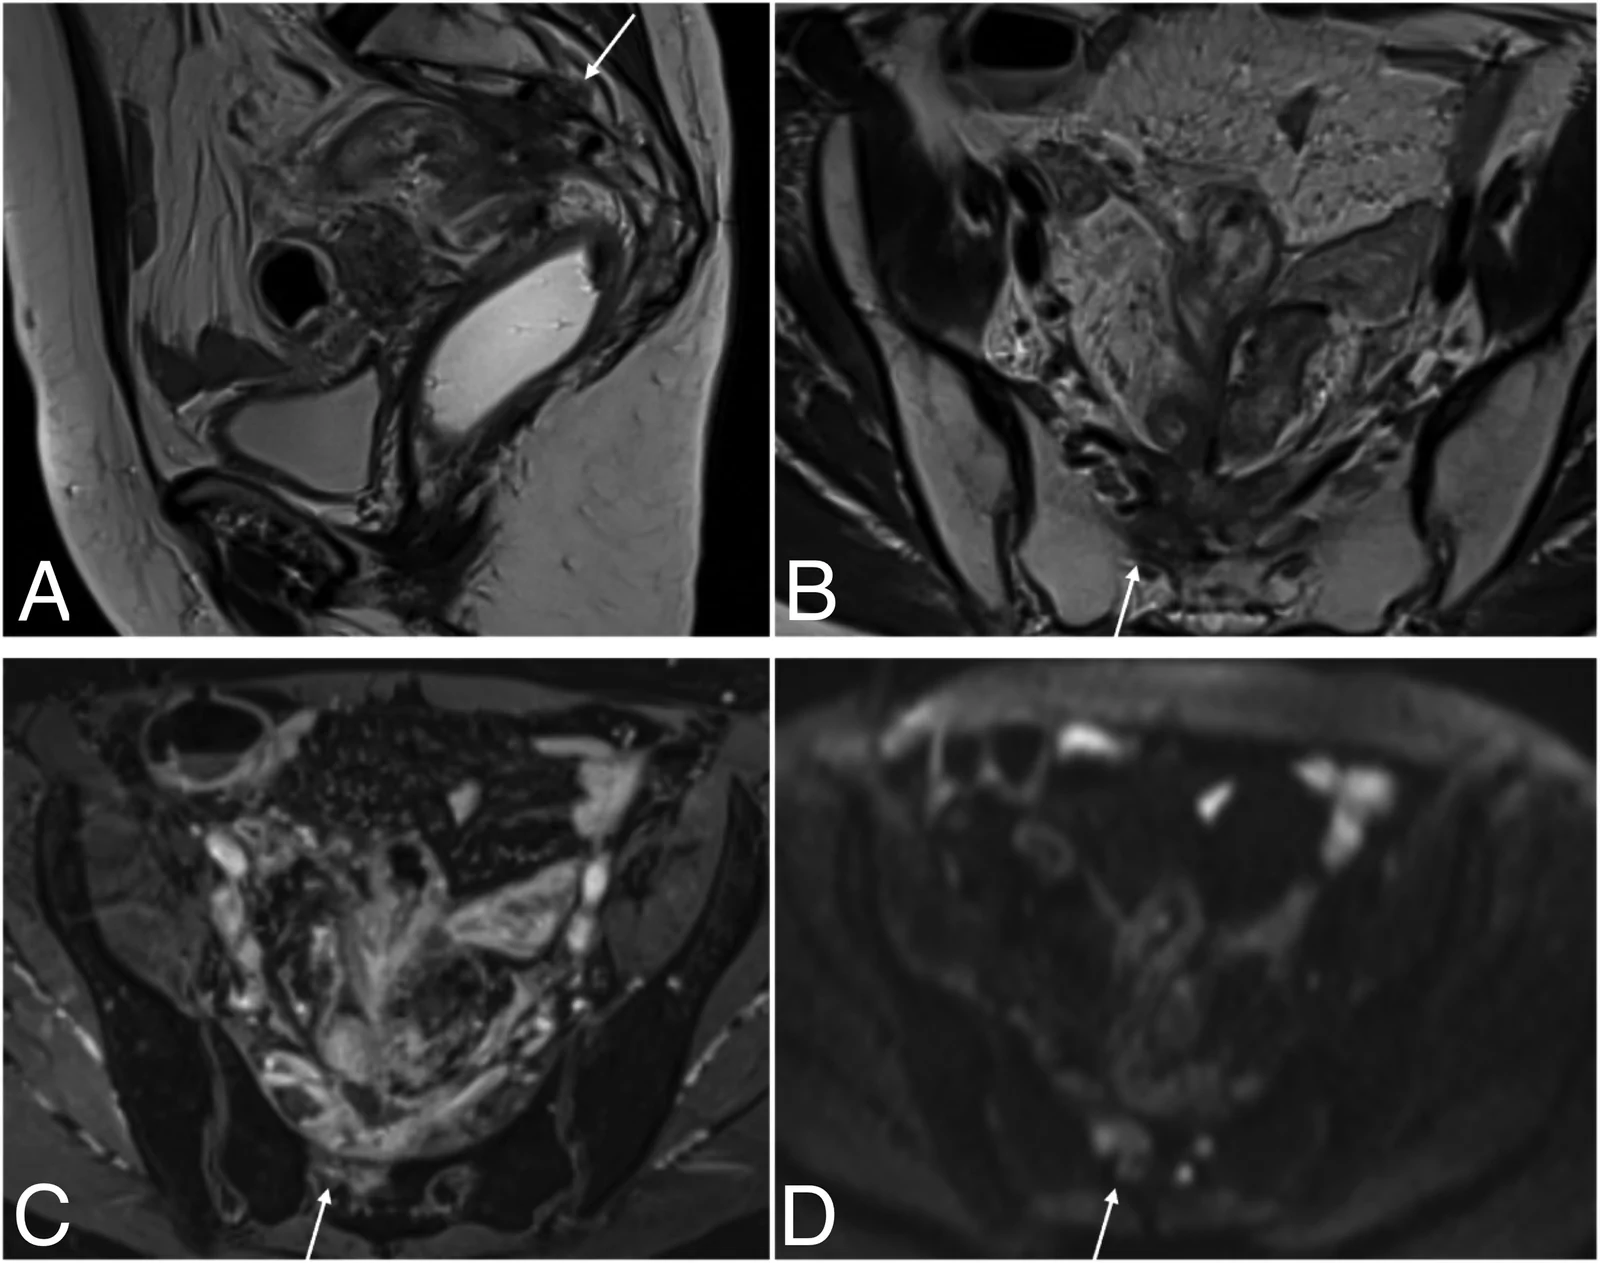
Recurrent rectal cancer with osseous extension into S2-S3 vertebrae on sagittal (A) and axial (B) T2-weighted images, confirmed by post-contrast T1 (C) and DWI (D). Key point: high sacral involvement (S2-S3) precludes en bloc exenteration.
